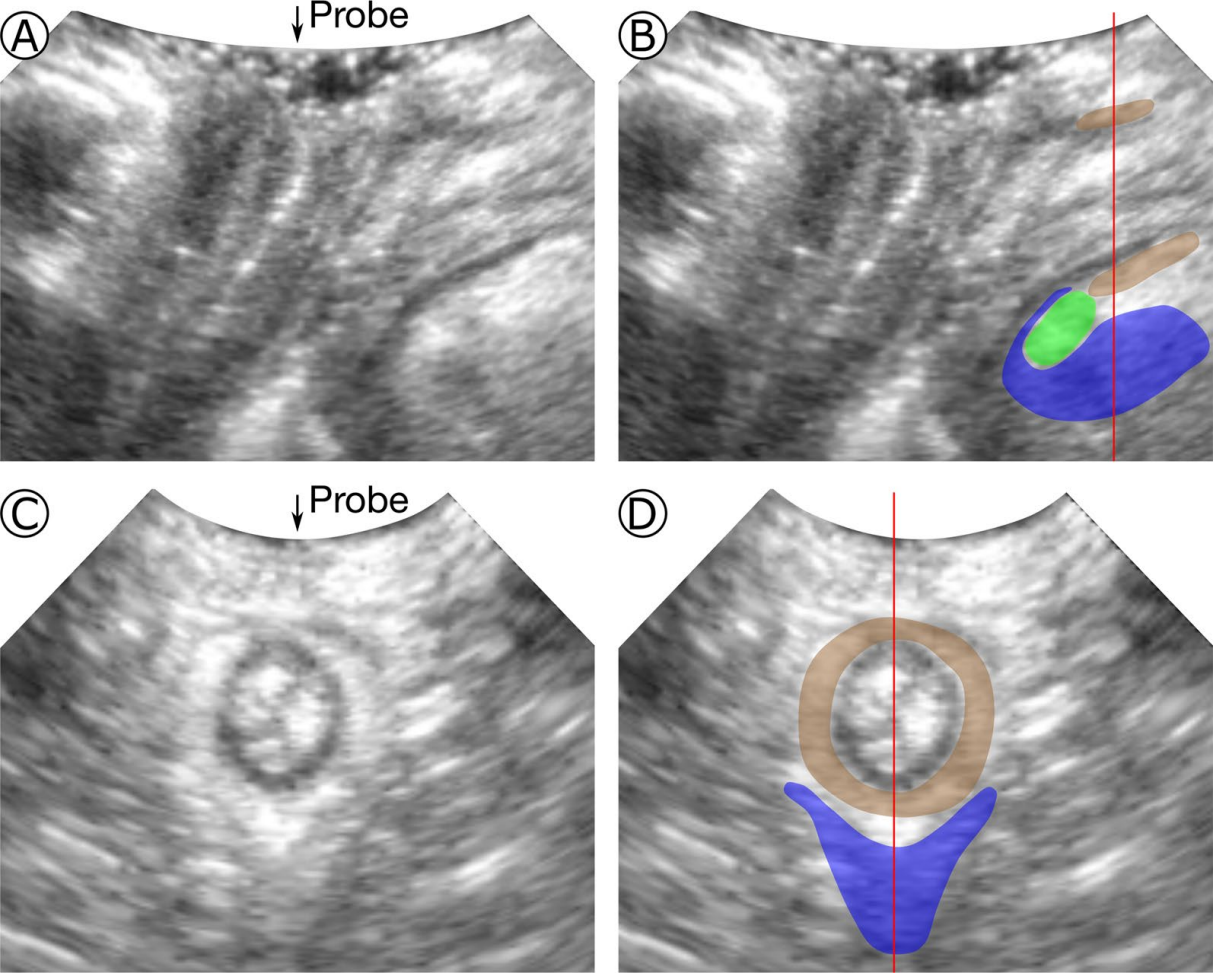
Midsagittal (A and B) and coronal (C and D) plane, without and with segmentation. In B, the C shape of the iliococcygeal muscle (ICM) (blue) surrounding the puborectal muscle (PRM) (green); in D, the round shape of the external sphincter (brown). The red line in B shows the position of the coronal plane and in D, the position of the midsagittal plane (A and B). The ultrasound probe position with respect to the images is indicated by the arrows
